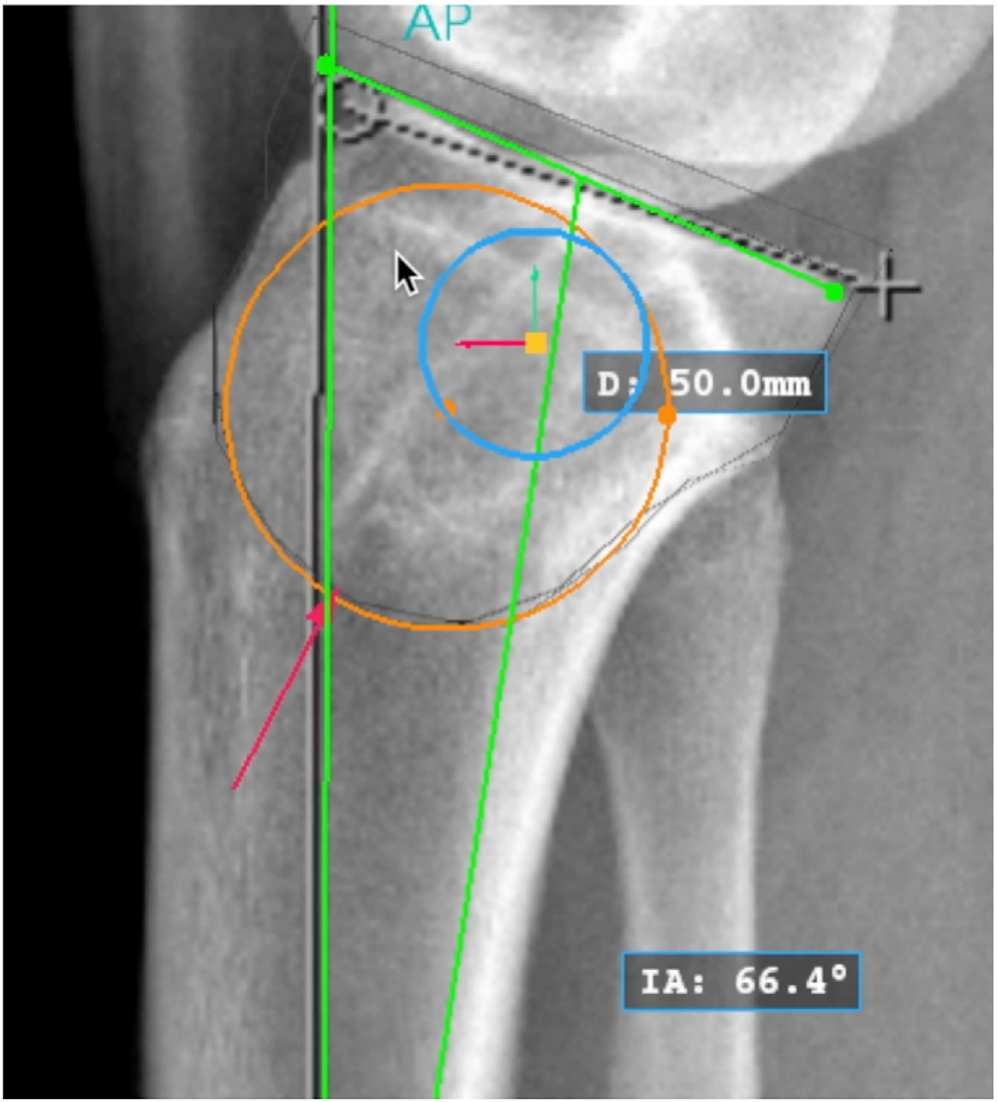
A lateral radiograph of the right knee shows the osteotomy center of rotation just above the proximal tibial-fibular joint, identified by the yellow dot. The osteotomy radius of rotation is identified by the blue circle, which subtends the center of the proximal tibia (orange dot at the center of the orange circle). The sagittal slope is measured using the anatomic axis (vertical green line with red arrow) and medial tibial plateau (IA: 66.4°). (D: 50.0mm) is the diameter of the best-fit circle. (D, diameter; IA, posterior tibial slope measure.)
